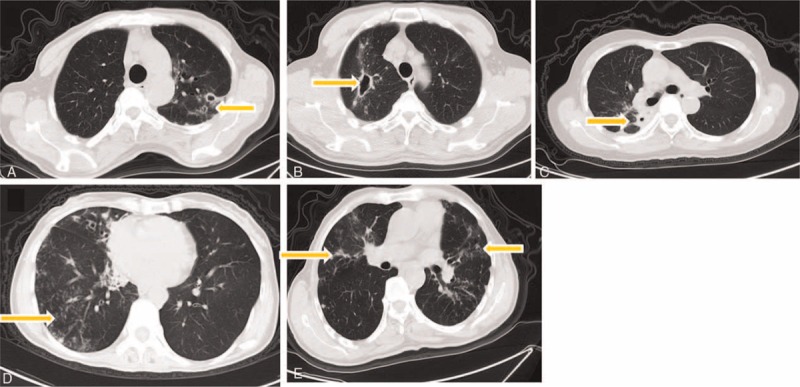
CT images of patients infected with *M. abscessus* (A-type) and *M. massiliense* (M-type). (A) Thin-walled small cavity in A-type patients’ CT scan, (B) thin-walled big cavity in A-type patients’ CT scan, (C) thick-walled small cavity in A-type patients’ CT scan, (D) tree-in-bud pattern in M-type patients’ CT scan, (E) patchy in M-type patients’ CT scan.
